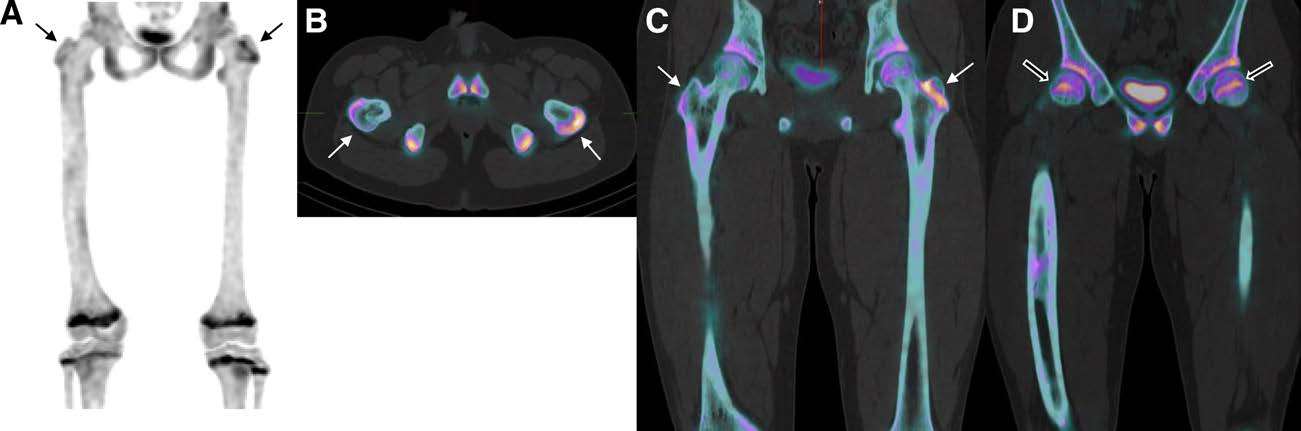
Follow-up bone SPECT/CT conducted 9 months after the initial bone SPECT/CT. Differences in the growth plate radioactivities between the proximal femurs became wider. Arrows show radioactivity in the growth plates of the greater trochanters, while empty arrows indicate those of the femoral heads (A: MIP image; B and C: axial and coronal fusion images of the greater trochanters; D: coronal fusion image of the femoral heads).
